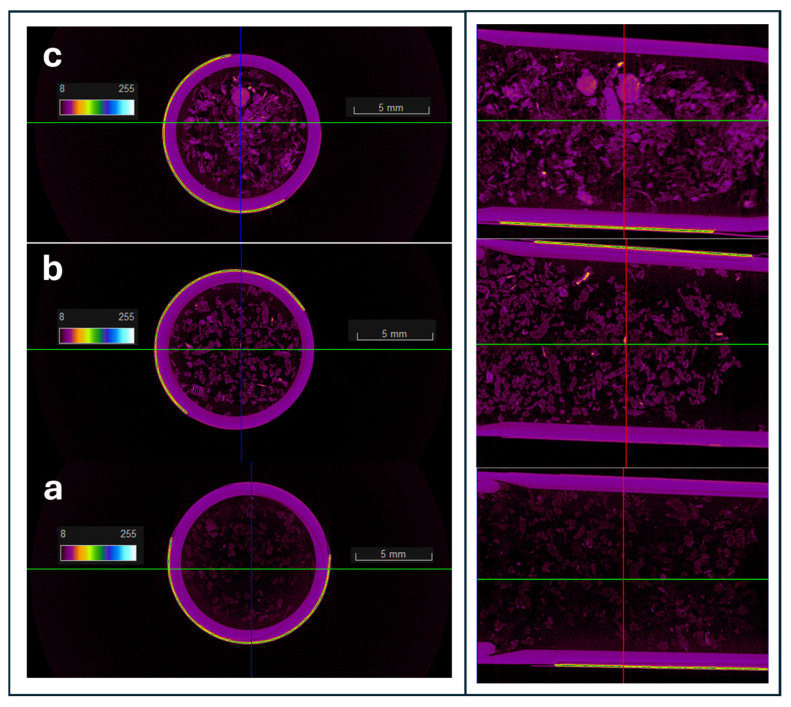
Microtomography CT images of: (a) EG; (b) EG/OD; and (c) EG/CNTs/OD. Green line is coronal (COR), blue line is sagittal (SAG) and red line is transverse (TRA) cross-sections of the samples.
